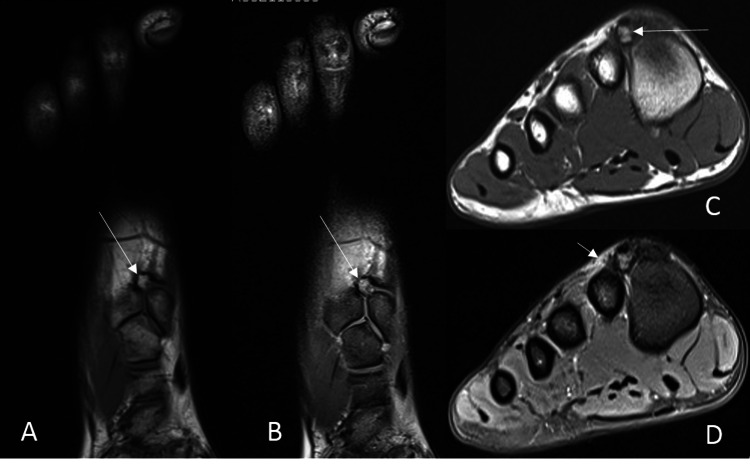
Tarsal os styloideum. MRI of the right foot. Coronal proton-density (PD) images without (A) and with (B) fat suppression. Transverse T1 (C) and PD fat-suppressed (PDFS) (D) images demonstrate bone fragments at the dorsal aspect between the first and second tarsometatarsal joints (long arrows). On sagittal images (not shown), the fragment was not visible owing to the slice thickness limitation. Note the high signal intensity of the fragment on PDFS images (B, D) indicating bone marrow edema of the fragment. Also note the increased PDFS signal (short arrow in D) indicating possible soft-tissue edema, although it could also be attributed to the incomplete fat suppression, which is possible in this location.
